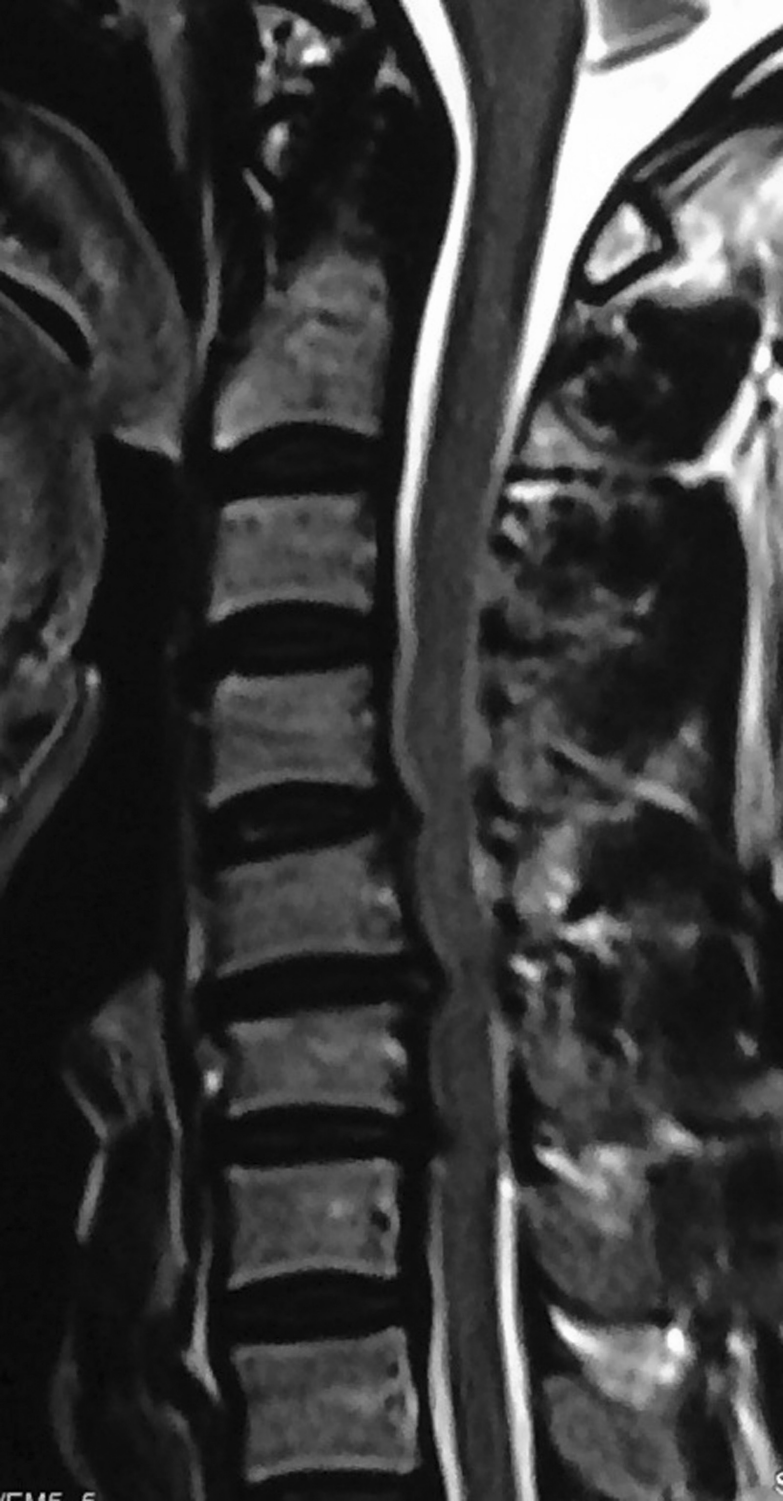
Case Example of a Three-Level ACDF Using Stand-Alone PEEK Cages A 52-year-old man presented with complaints of neck pain, radiculopathy, and cervical spondylotic myelopathy with a sagittal T2 weighted MRI demonstrating three cervical disc herniations.
